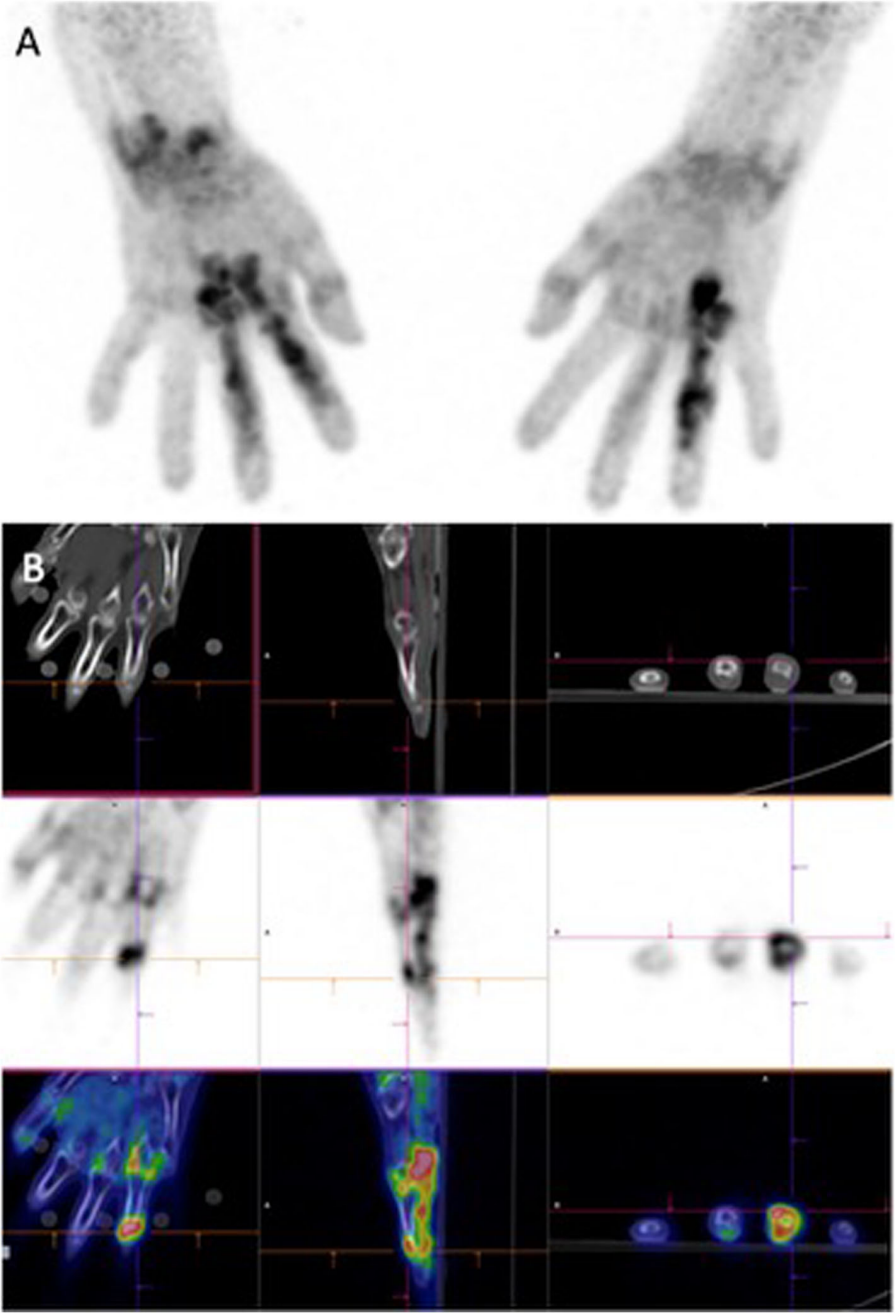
Extensive inflammatory changes in several joints of the hand, along with tenosynovitis. a: MIP (maximum intensity projection) of the [^18^F] FDG PET image. b: CT (upper row), PET (middle row) and fused PET/CT images (lower row) in the coronal, sagittal and transaxial views, respectively. [^18^F] FDG PET/CT: ^18^F-fluorodeoxyglucose positron emission computed tomography
